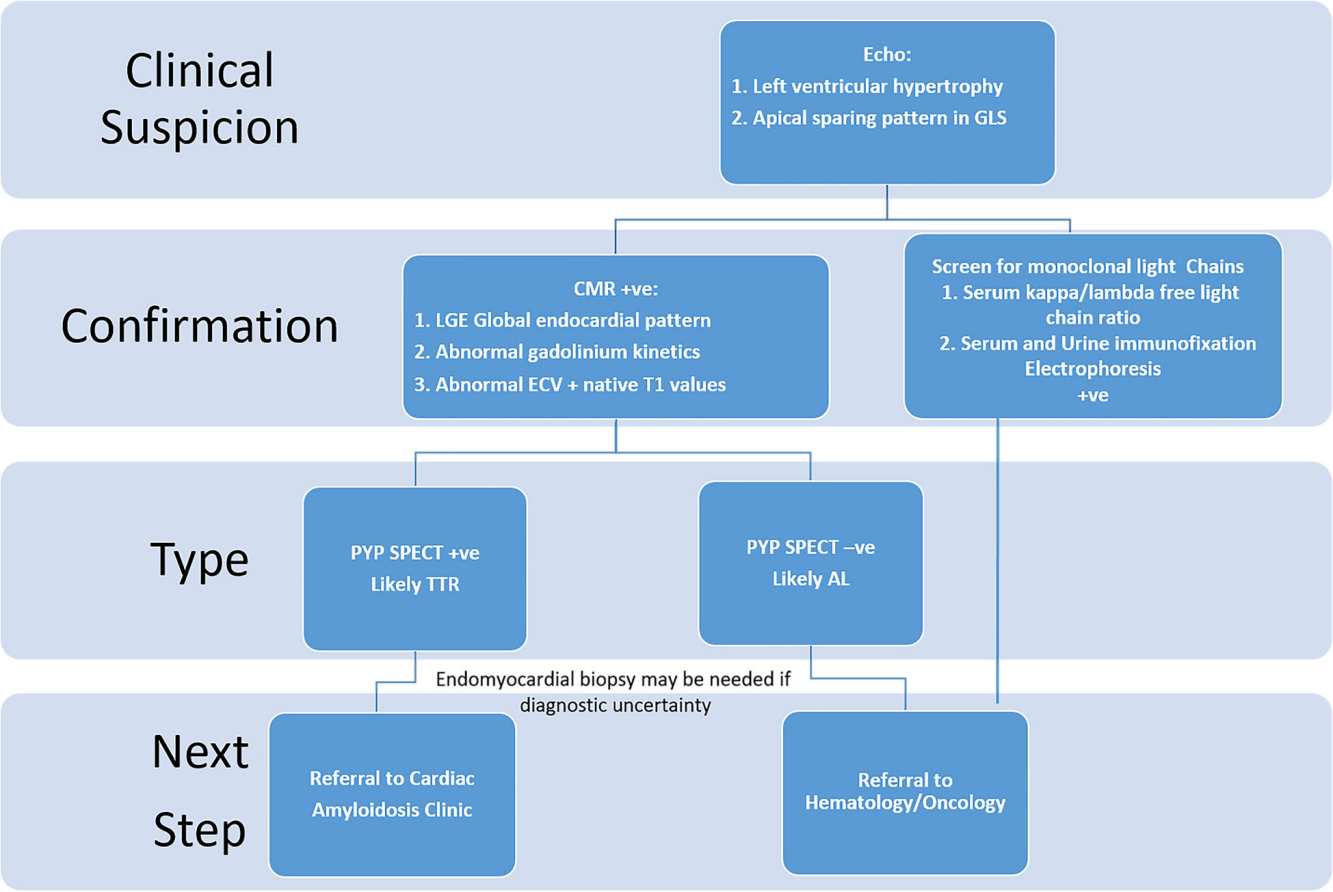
Diagnostic algorithm for workup of clinically suspected cardiac amyloidosis. Modified from Agha AM, et al. Role of cardiovascular imaging for the diagnosis and prognosis of cardiac amyloidosis. Open Heart. 2018 Sep 26;5 (2):e000881.doi:10.1136/openhrt-2018-000881. eCollection 2018. review. AL,light chain amyloidosis; ATTR,abnormal transthyretin; CMR, cardiovascular magnetic resonance; DPD, 3,3-diphosphono-1,2-proporanodicarboxylic acid; ECV, extracellular volume; GLS, global longitudinal strain; LGE, late gadolinium enhancement; PYP, pyrophosphate; SPECT, single photon emission CT [4]
